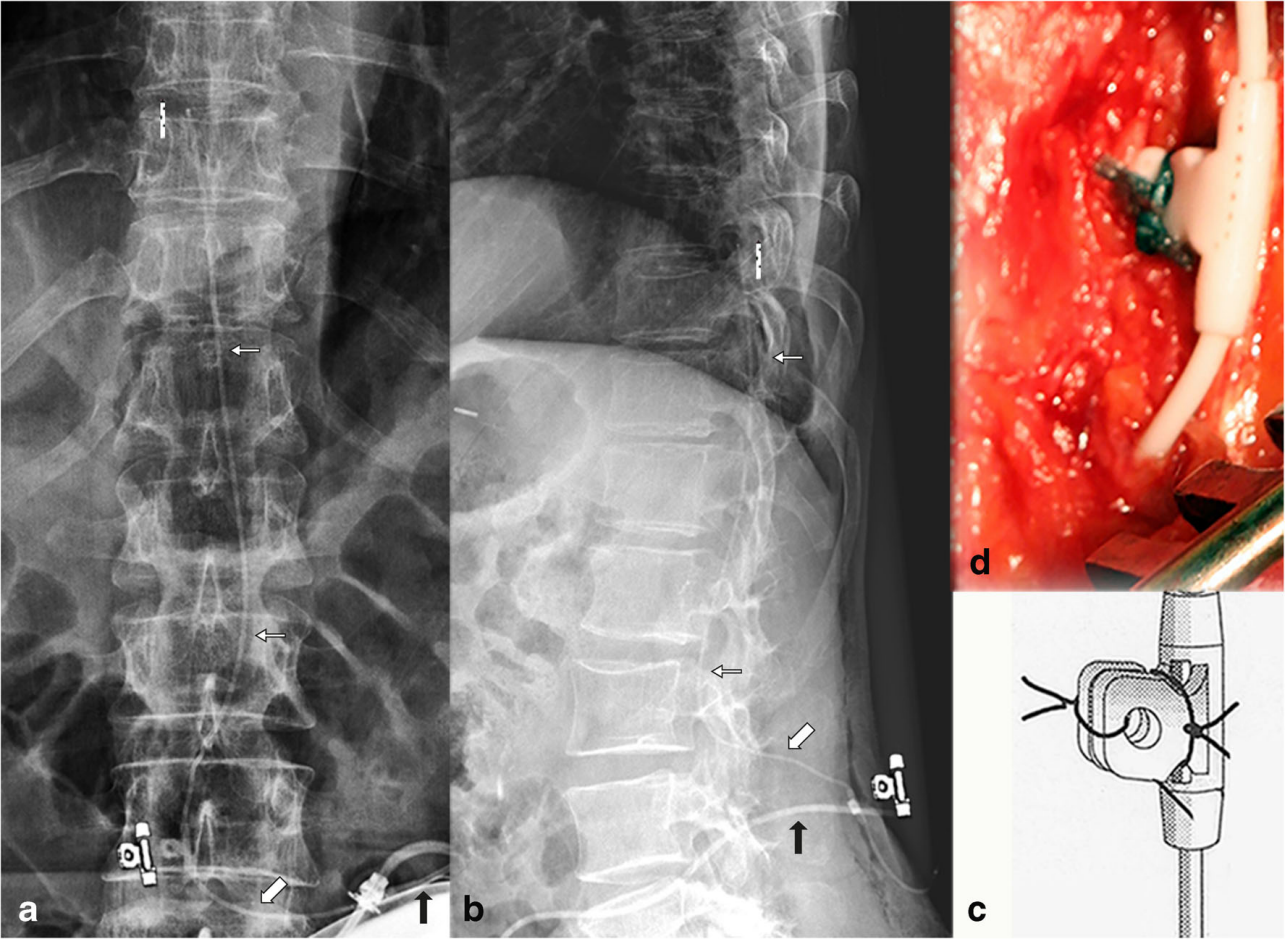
Anterior-posterior (a) and lateral (b) plain radiography of the lumbar spine, in vivo image (d) and artist rendering (c) of the typical *folded* fixation anchor (with *anchor symbol*), large diameter catheter pump segment (*black arrow*), small diameter outside spinal canal (*thick white arrow*) and intrathecal (*small white arrow*, *catheter end symbol*) segment of the 8731SC catheter
